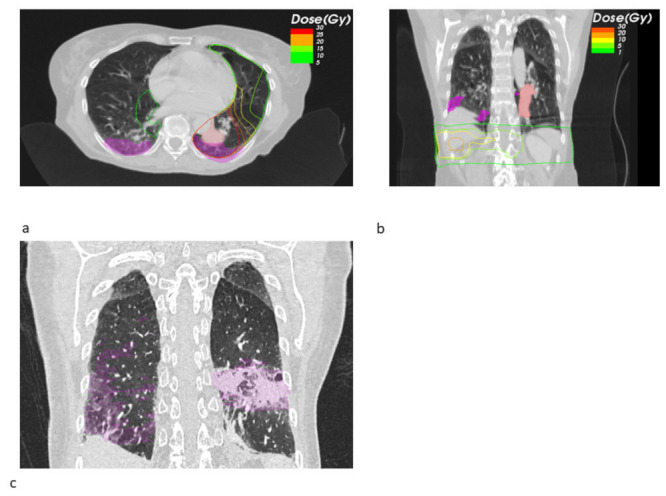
Transversal radiotherapy (RT) planning CT slices showing the sum EQD2 isodoses and pneumonitis contours (magenta) and GTV contours (light red) for a SBRT+ ICB case (a). This patient received simultaneous liver metastasis SBRT. The isodoses of the original treatment plan show a negligible lung dose (b). Figure (c) shows an exemplary sagittal slice from the follow-up CT showing the bilateral pneumonitis and its contours (magenta).
